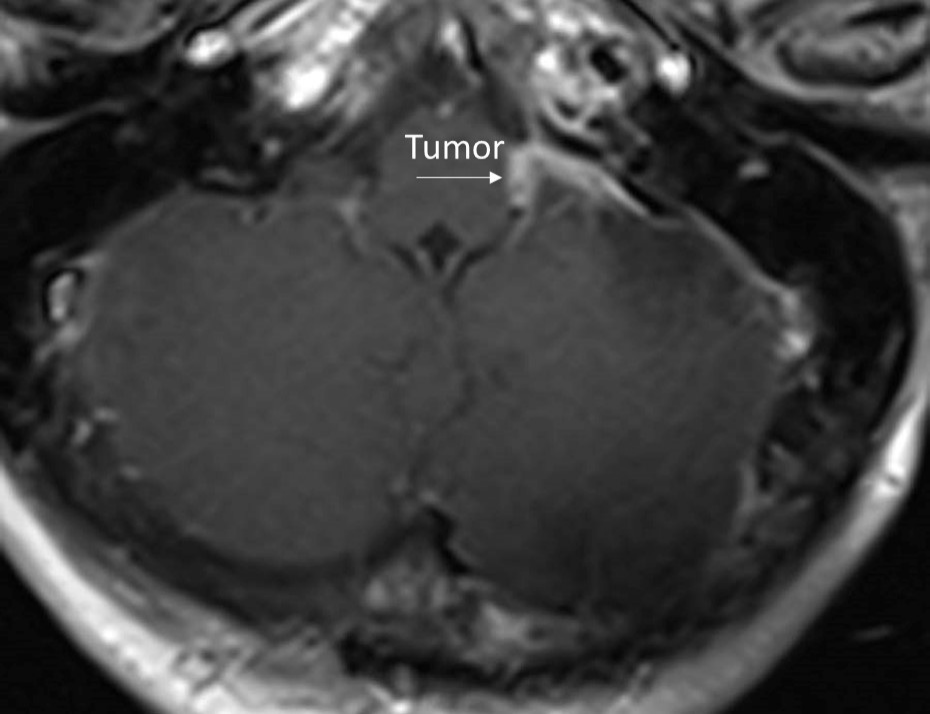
Postoperative MRI of Case 2 Postoperative MRI with contrast depicting a near total gross resection of the jugular foramen lesion via a retrosigmoid approach.
